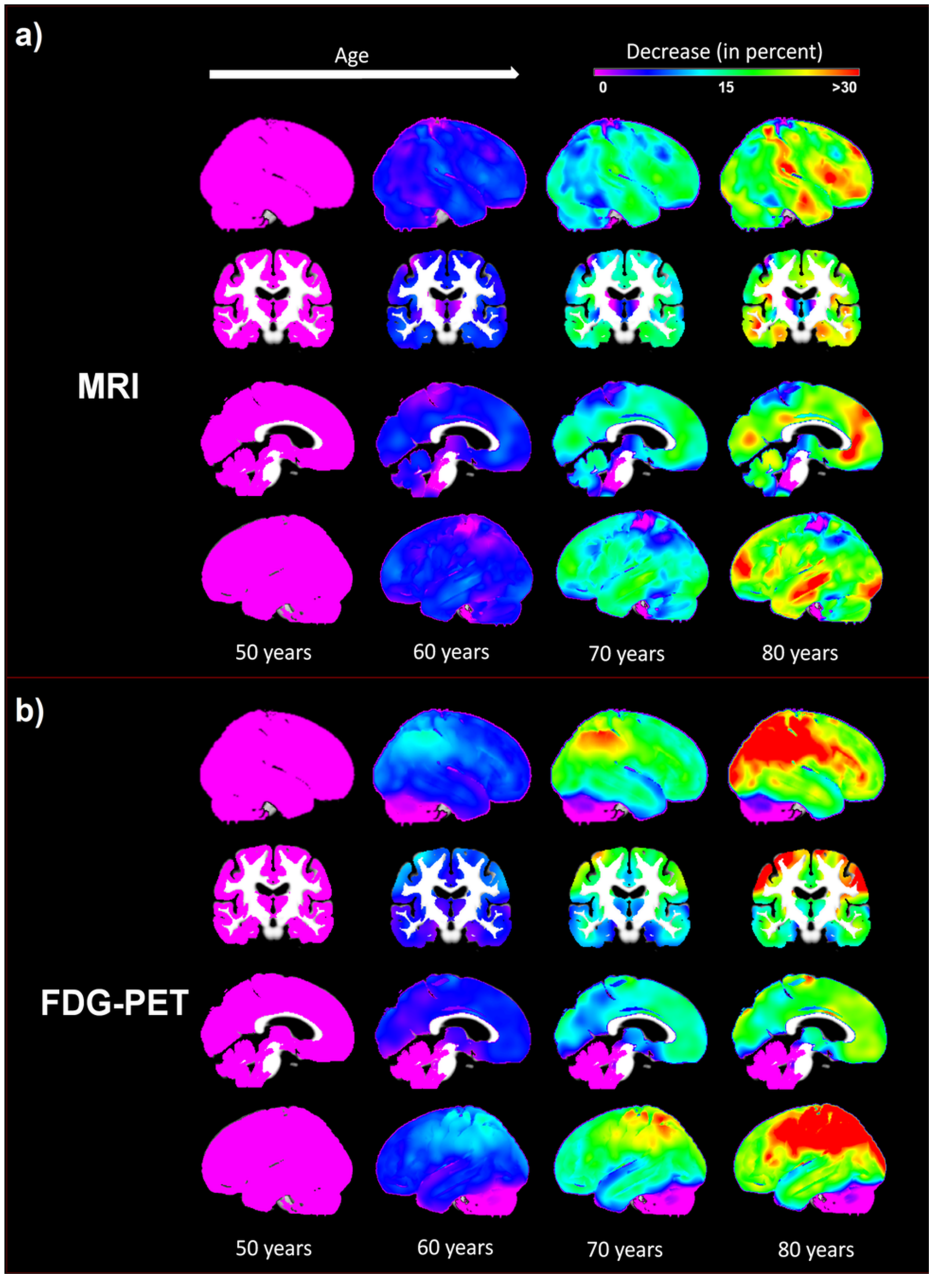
Healthy aging related changes observed in MRI (a) and FDG-PET (b) considering the expression of GM volume and glucose metabolism at the age of 50 years as baseline. FDG-PET [18F]fluorodeoxyglucose positron emission tomography, GM grey matter, MRI structural magnetic resonance imaging.
